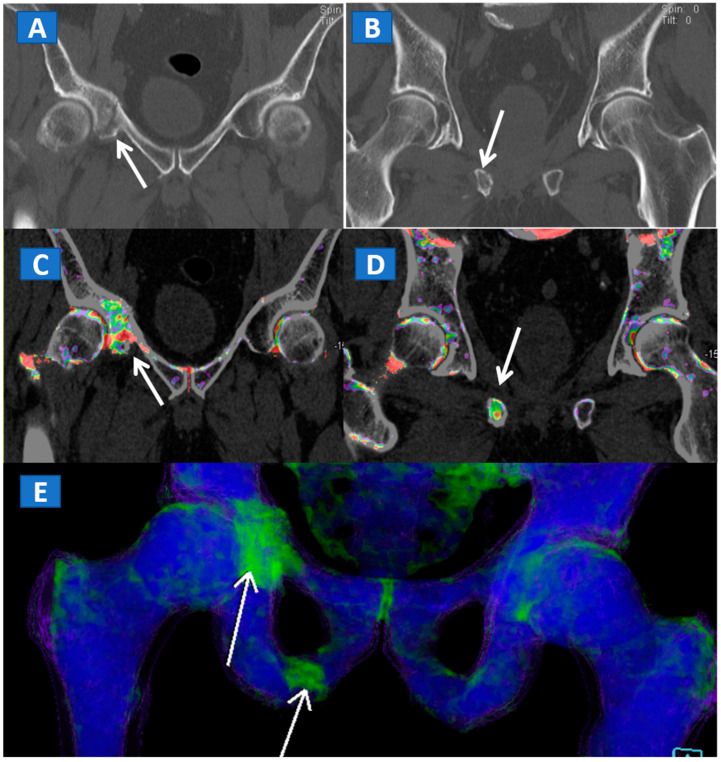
A 61-year-old female with non-displaced fractures of the right iliac and pubic bones. On the standard coronal 1 mm CT images (A,B) the iliac fracture is well depicted (arrow in (A)), whereas the pubic fractures could be easily missed (arrow in (B)). The presence of BME on the corresponding 1 mm coronal reconstructed DECT images (C,D) confirms the non-displaced fractures. The 3D coronal DECT map (E) yields a clear picture, depicting the fractures of the right side and ruling out the presence of BME on the left side.
